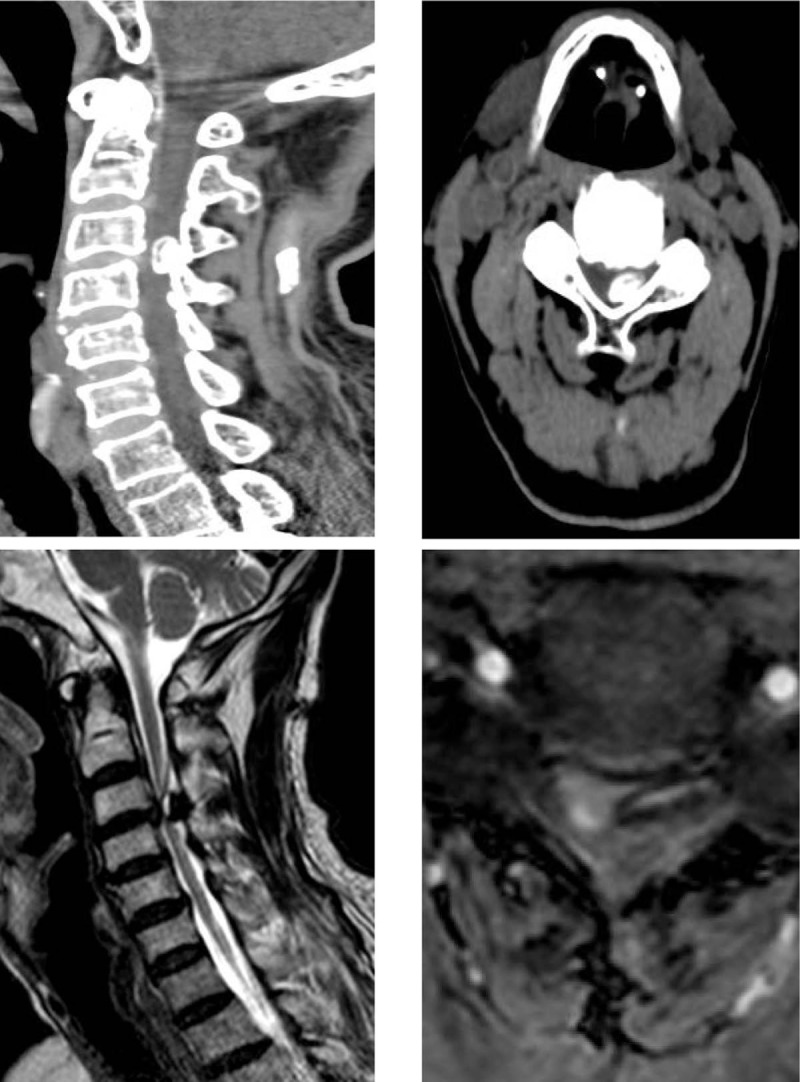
Preoperative Imaging of the cervical spine. (a, b) Axial and sagittal CT showed a circular high density mass in the left posterior part of the C3/4 spinal canal. (c, d) Axial and sagittal MRI showed a larger compression mass at C3/4 levels.
